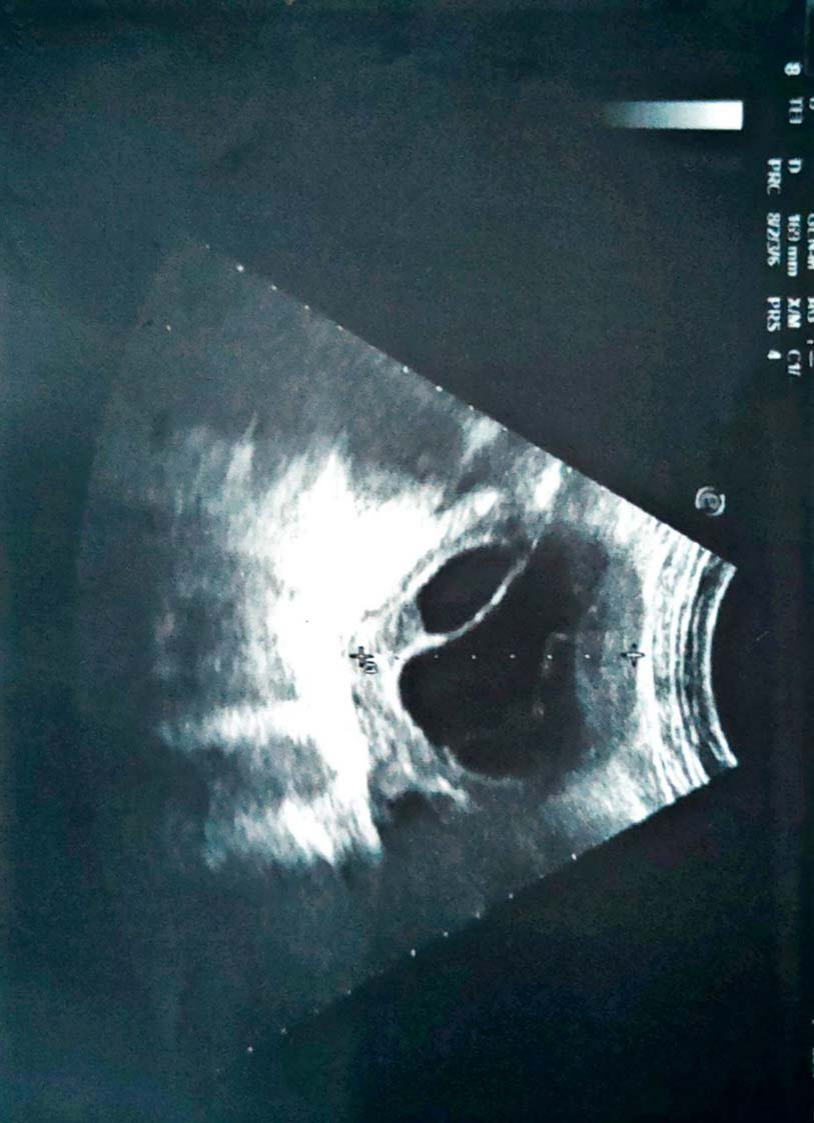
An ultrasound showing a well circumscribed, multivesicular, fluid-containing cyst.
